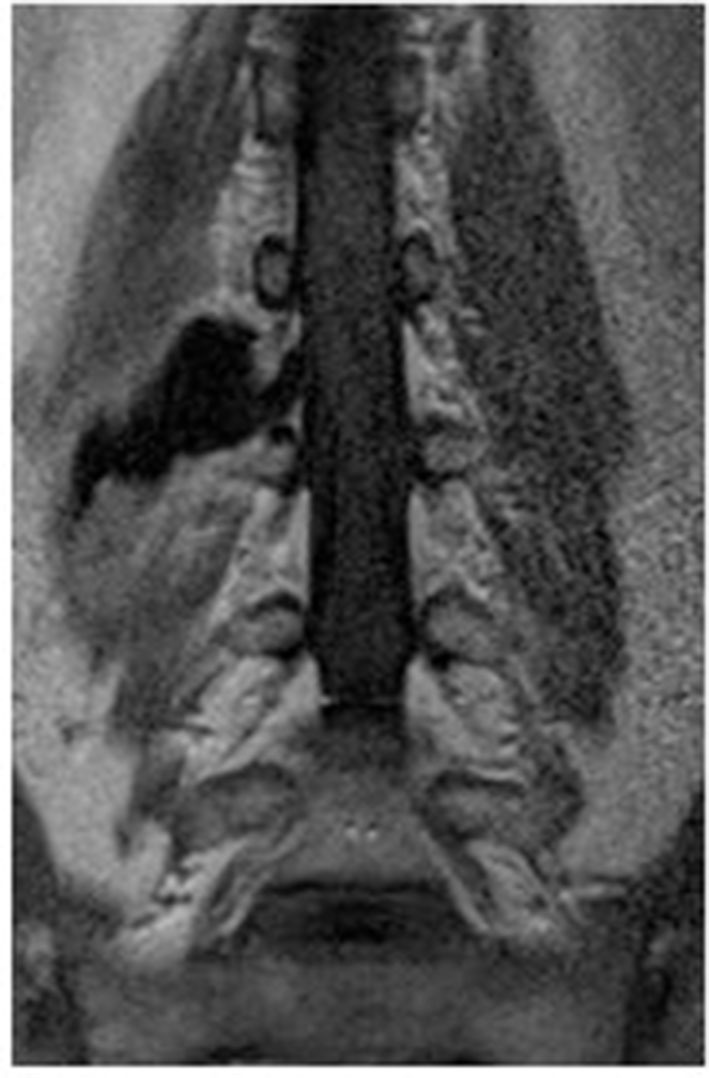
MRI of the lumbar spine with contrast after the surgery.
